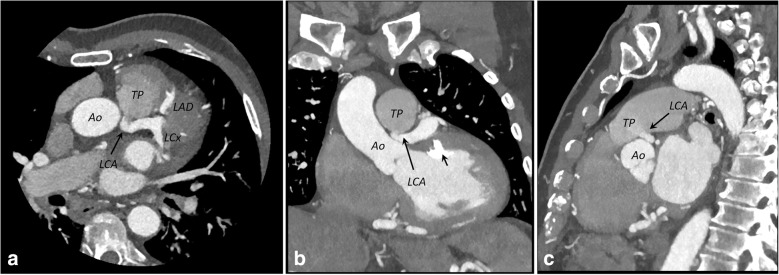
a–c Axial (a), coronal (b), and sagittal (c) maximum-intensity projection (5 mm) coronary computed tomographic angiographic images depicting the origin of the left coronary artery (LCA) (*arrows*) from the pulmonary trunk (TP) and not from the Aorta (Ao). Several calcifications are noted at the papillary muscle (*short arrow* in b), resulting from chronic ischemic changes. *LAD* Left coronary artery, *LCx* Left circumflex coronary artery
